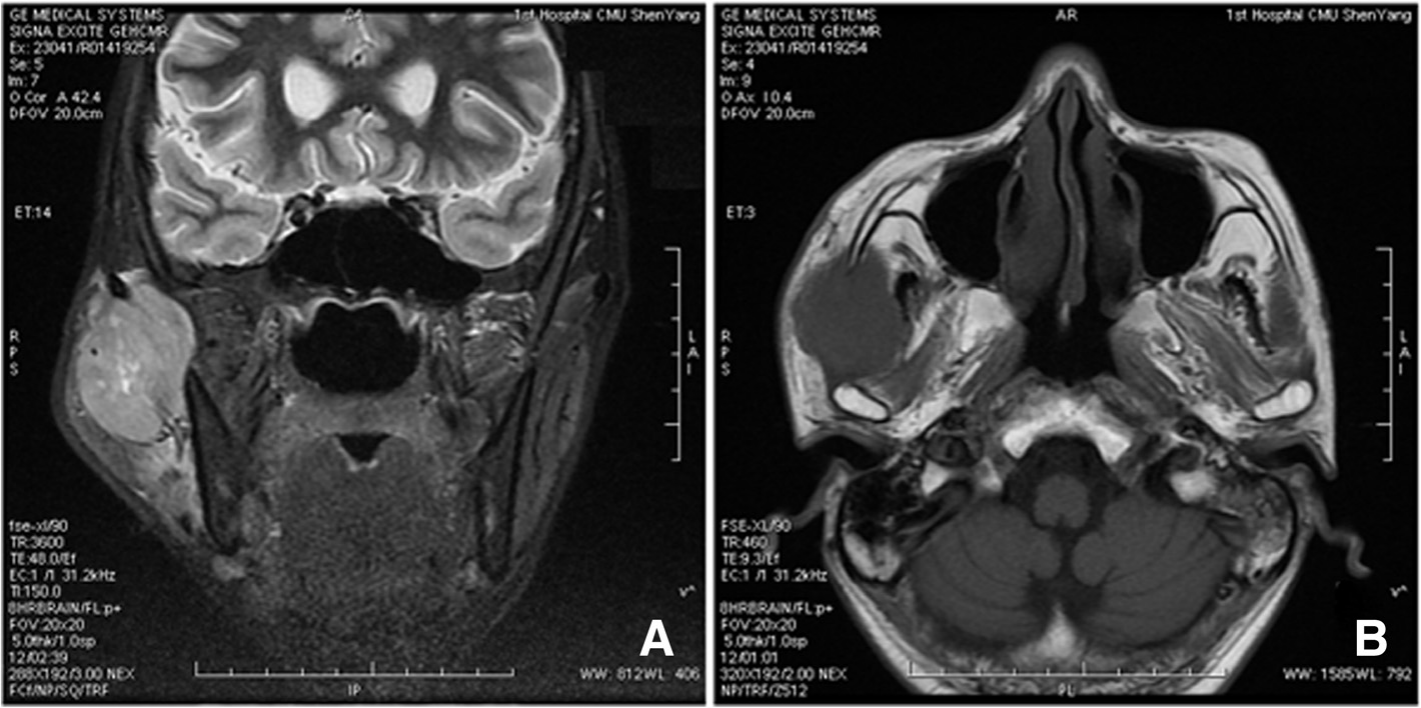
Magnetic resonance imaging revealing the tumor. A and B, Magnetic resonance imaging revealed an oval mass about 5.2× 3.1 cm in the right lower masseter. The tumor was relatively well circumscribed.
